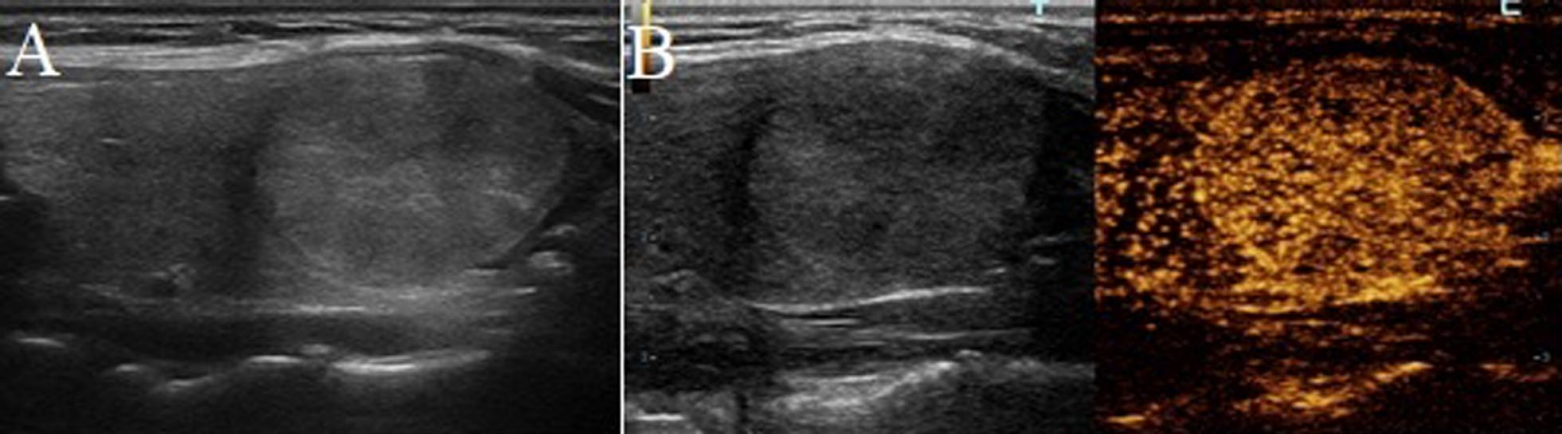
A follicular adenoma in a 53-year-old woman. A Greyscale ultrasound showed that there was a halo surrounding the thyroid nodule. B Contrast-enhanced ultrasound revealed regular high-enhancing ring (at the 24th second after the injection of contrast)
